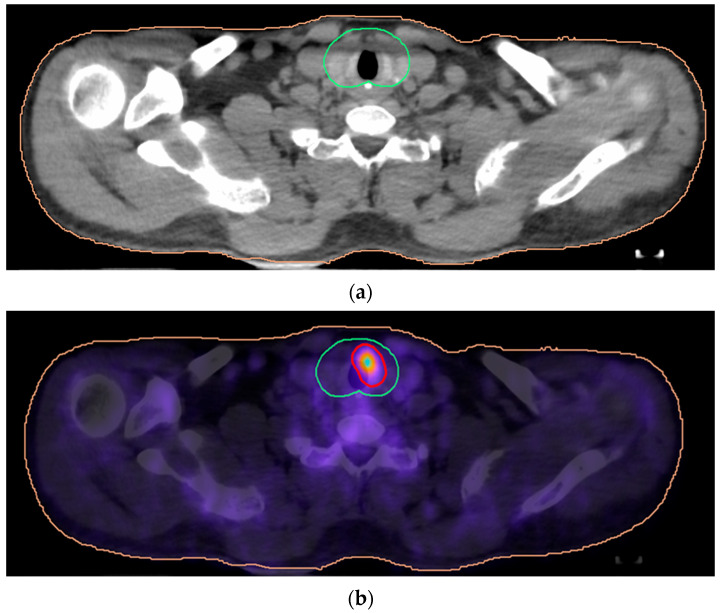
Examples of improvement in primary tumor coverage after PET/CT-based radiotherapy planning by adding a boost. Contouring a primary tumor area (green line; PTV1) based on CT treatment planning (a). A PTV4 area was added to extend primary tumor coverage by including a boost based on PET/CT-detected paratracheal involvement (b).
